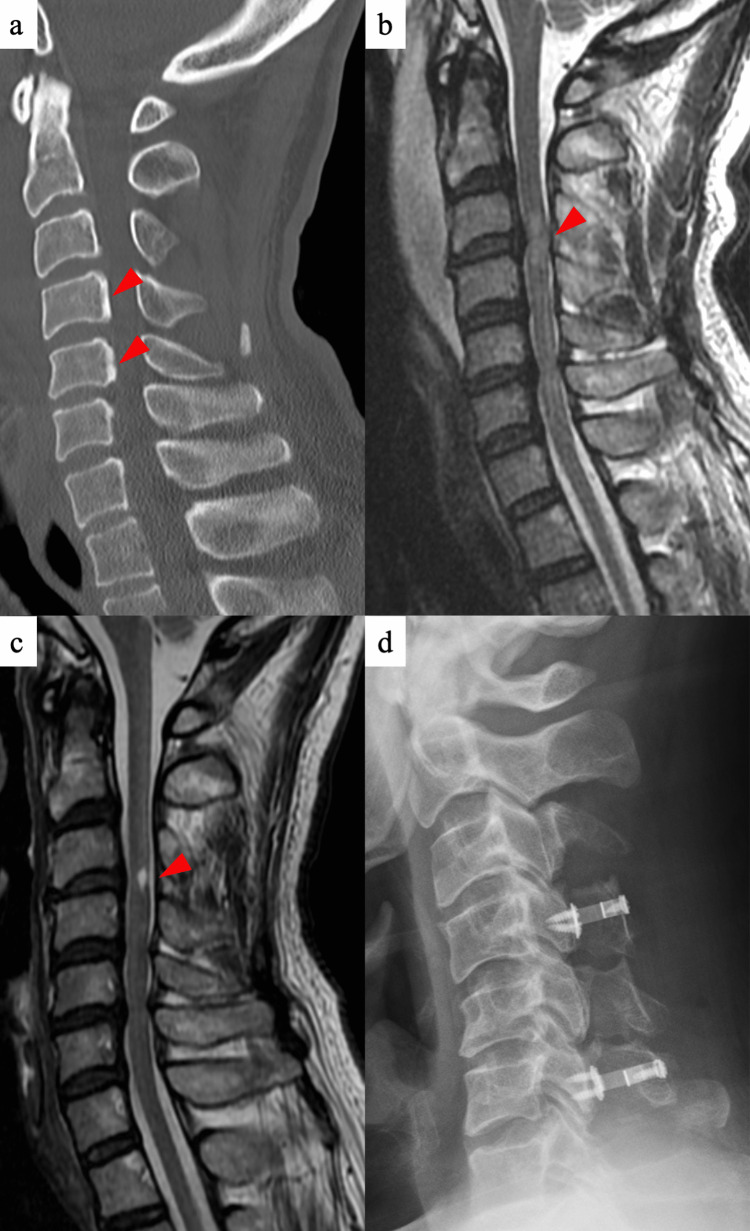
Imaging findings before and after surgery (A) Computed tomography scan after emergency transport; a narrow canal between C4 and C5 (11 mm), ossification of the posterior longitudinal ligament of the segmental type on the posterior walls of C4 and C5, and no fractures. (B) T2-weighted magnetic resonance imaging scan after emergency transport. The spinal cord compression rate was 21%. (C) T2-weighted magnetic resonance imaging scan at eight months after injury. Edema was reduced, and the spinal cord compression rate was 16%. (D) X-ray findings after decompression surgery. The spinal canal enlargement rate was 173%.
